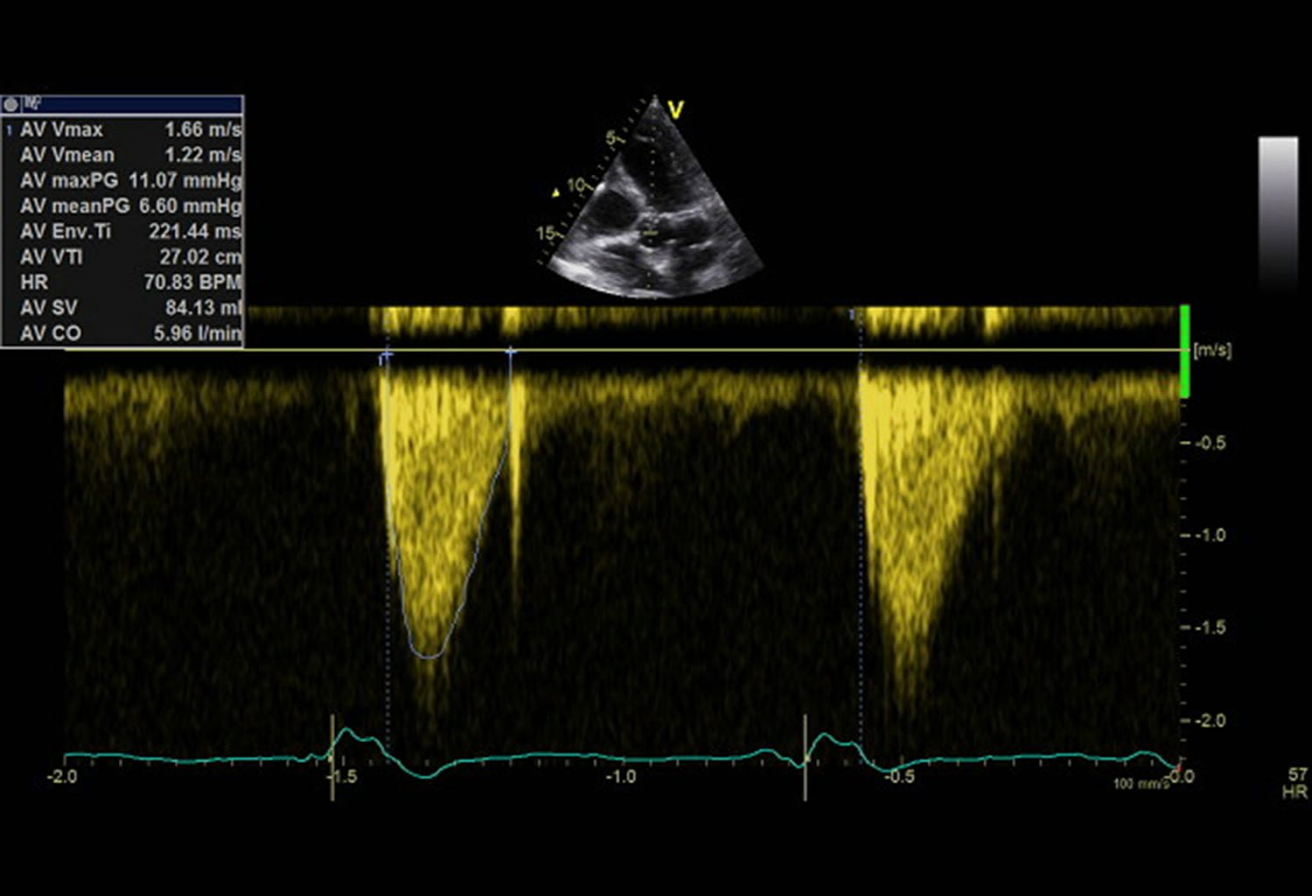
Continouous wave Doppler across the aortic valve to measure the velocity time integral
